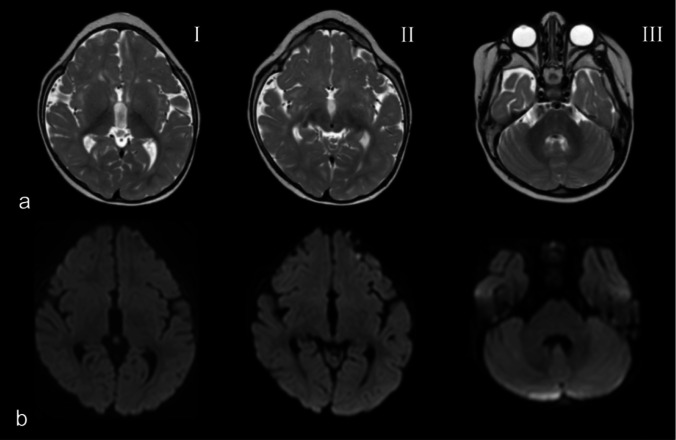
Brain magnetic resonance imaging of the 17-month-old boy with developmental and epileptic encephalopathy, trigonocephaly due to metopic suture synostosis, and a pathogenic *POLR2A* variant, performed 8 months after gradual discontinuation of Sabril® (vigabatrin). (*a, b*) Axial T2-weighted turbo spin-echo (*a*) and axial diffusion-weighted (*b*) images without contrast administration demonstrate normal signal of the globi pallidi (*I*), subthalamic nuclei (*II*), and central tegmental tracts (*III*), with complete resolution of the bilateral and symmetrical deep gray matter and brainstem signal abnormalities, without residual sequelae
